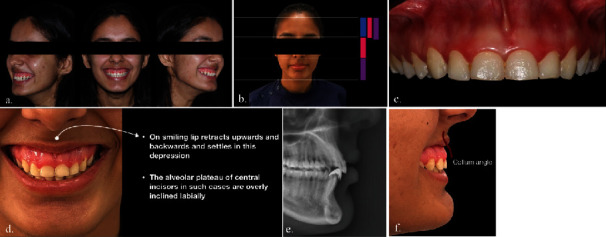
(a) Preoperative front and lateral profile. (b) Extraoral examination: face analysis. (c) Intraoral examination: shorter clinical crown. (d) Lip analysis. (e–f) Radiographic examination: collum angle (the supplementary angle of the crown-root angle).
